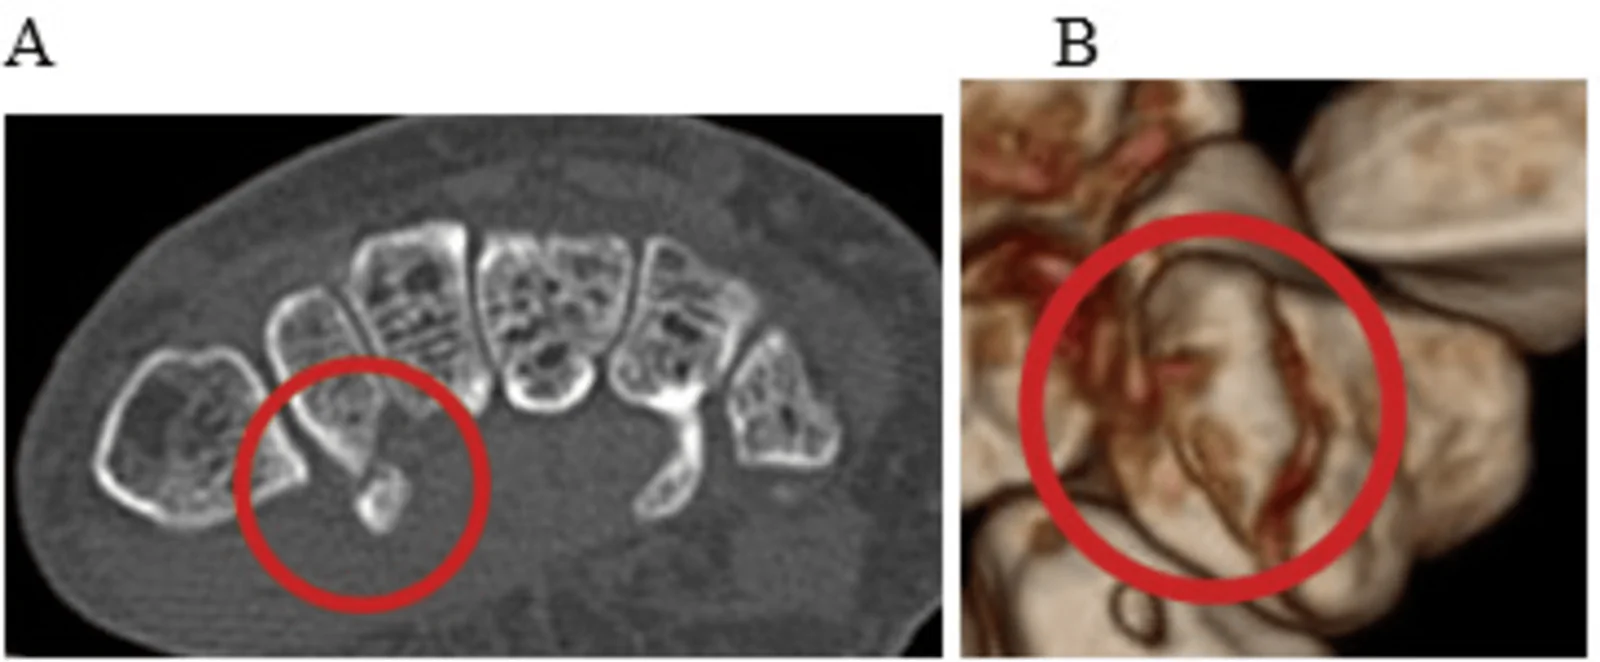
Case 2: Initial CT findings. Axial (A) and three-dimensional (B) CT images showing a fracture line in the trapezial tubercle (red circles).
